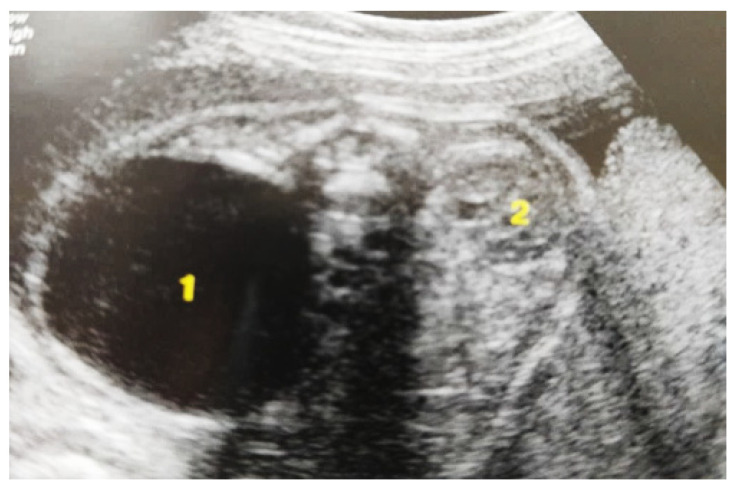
Ultrasound at the 20th week of gestation. Hydronephrosis on the right. (1) Dilated pelvis on the right. (2) Uninjured renal parenchyma on the left.
